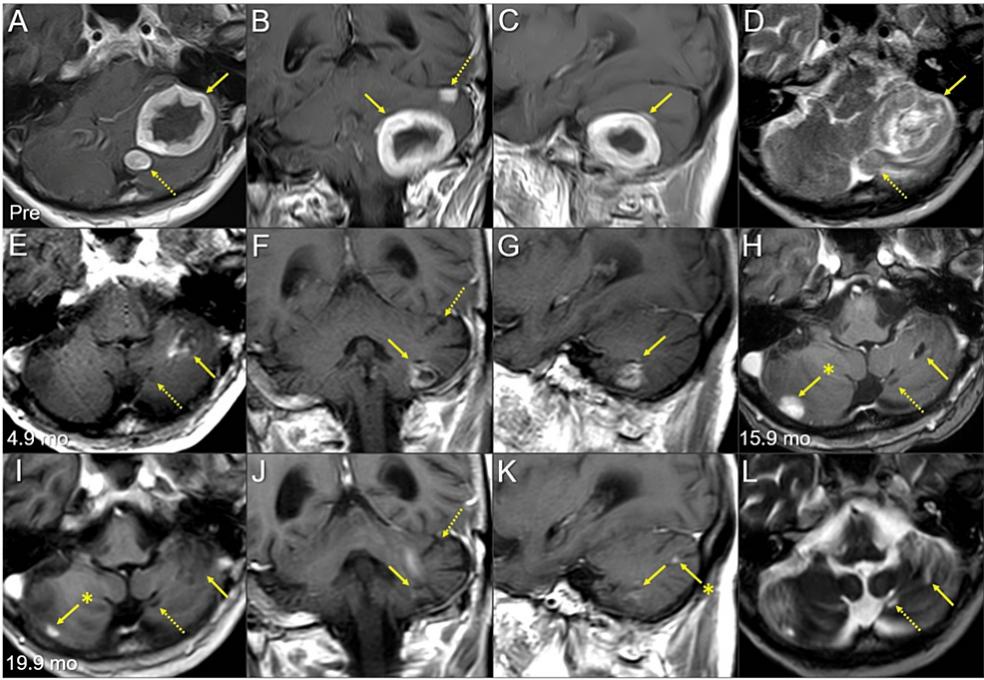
Magnetic resonance images of the posterior fossa lesions at the initial diagnosis and after whole-brain radiotherapy The images show contrast-enhanced (CE) T1-weighted images (WIs) (A-C, E-K); T2-WIs (D, L); axial images (A, D, E, H, I, L); coronal images (B, F, J); sagittal images (C, G, K); 13 days before (pre) the initiation of whole-brain radiotherapy (WBRT) (A-D); at 4.9 months (mo) after WBRT initiation (E-G); at 15.9 months (H); and at 19.9 months (I-L). (A-L) These images are shown at the same magnification and coordinates under co-registration and fusions. (A-D) A well-demarcated lesion with the dominance of the peripheral enhancement (3.8 cm in the maximum diameter, 17.7 cm^3^ in the volume) (arrows in A-C) on CE-T1-WIs is observed as the heterogeneous intensity mass (arrow in D) associated with mild perilesional edema on T2-WI. The lesion reaches the cerebellar surface at the ventrolateral and caudal sides and appears to extend beyond it in some areas. In the ipsilateral hemisphere, a 1.1 cm solid lesion (0.8 cm^3^) is located adjacent to the 3.8 cm lesion (dashed arrow in A, D), and a 0.9 cm lesion (0.3 cm^3^) is located less than 1 cm away from the 3.8 cm lesion (dashed arrow in B). (E-G) At 4.9 months, T2-WIs were unavailable. The large cerebellar lesion remarkably decreased in size, along with significant attenuation of the enhancing effect (arrows in E-G), and the two adjacent lesions (dashed arrows in E, F) regressed completely. (H) At 15.9 months, the large lesion was faintly enhanced at the periphery (arrow in H). The adjacent lesion is observed as the cavitary scar without enhancement (dashed arrow in H). A new enhancing lesion (arrow with an asterisk in H) appeared. (I-L) At 19.9 months, the enhancement of the large lesion was further attenuated (arrows in I-K), with the faint high-intensity scar (arrow in L) visible on T2-WI. None of the other two lesions showed local progression (dashed arrows in I, J, and L). The right cerebellar lesion regressed markedly (arrow with an asterisk in I). A new enhancing lesion (arrow with an asterisk in K) appeared.
